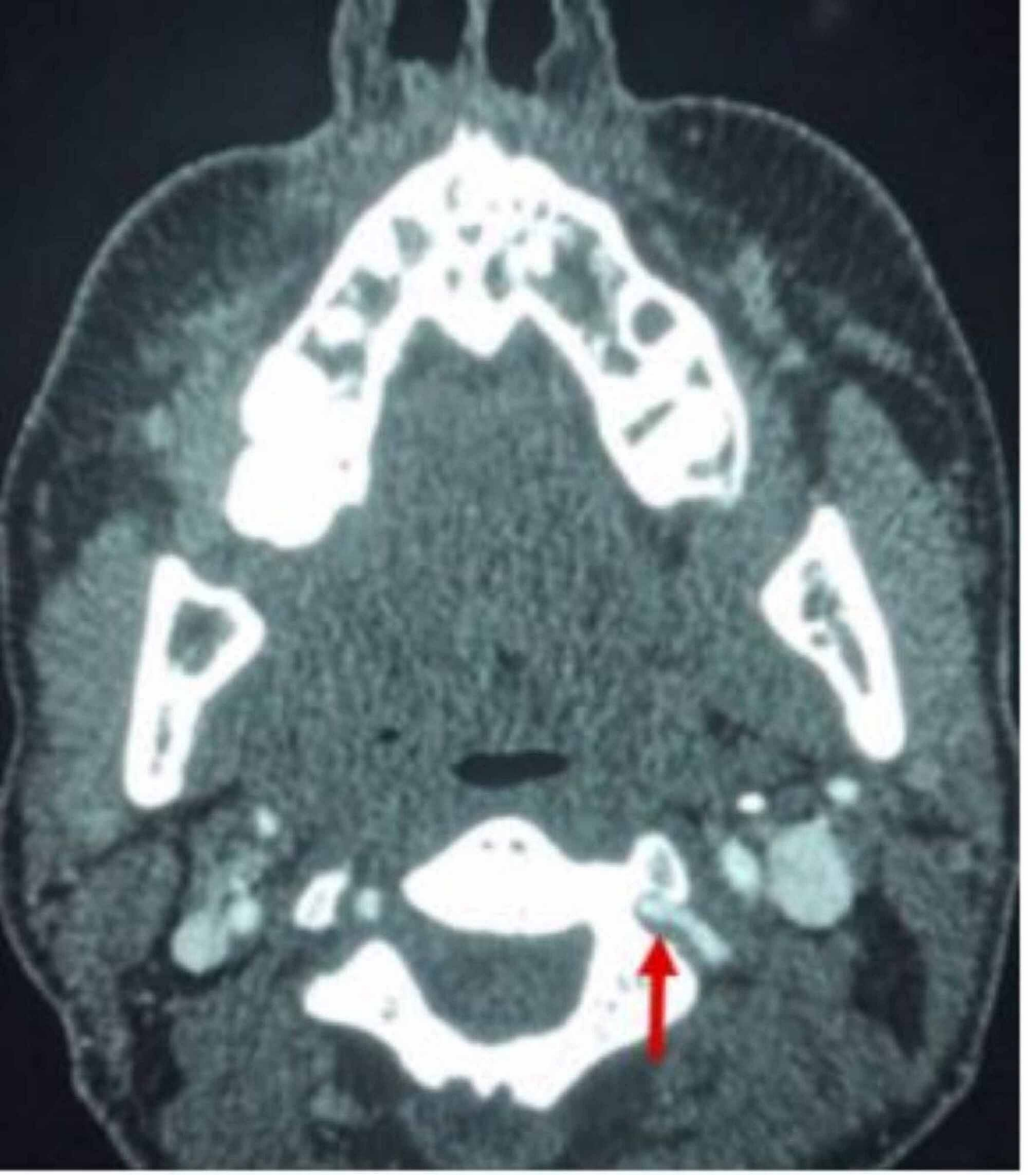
Left Vertebral Artery Intimal Flap Axial CT angiography (CTA) showing a patent intimal flap with possible thrombosis in the left vertebral artery (arrow).
